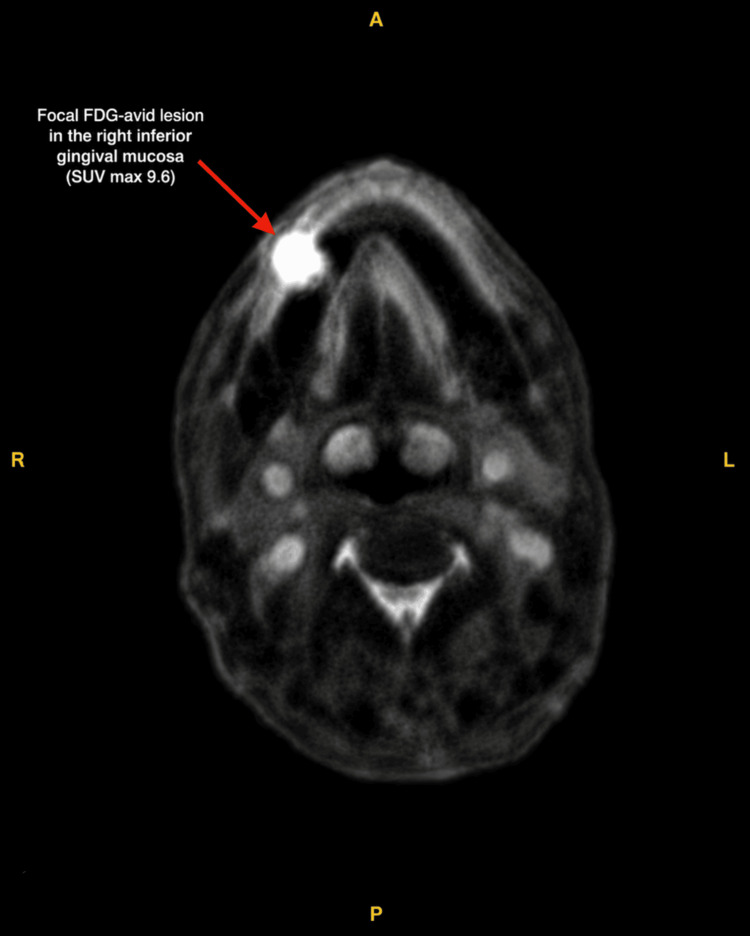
Positron emission tomography/computed tomography (PET/CT) showing a fluorodeoxyglucose (FDG)-avid primary gingival mucosal melanoma as a focal lesion involving the right inferior gingival mucosa (SUVmax, 9.6, red arrow).
